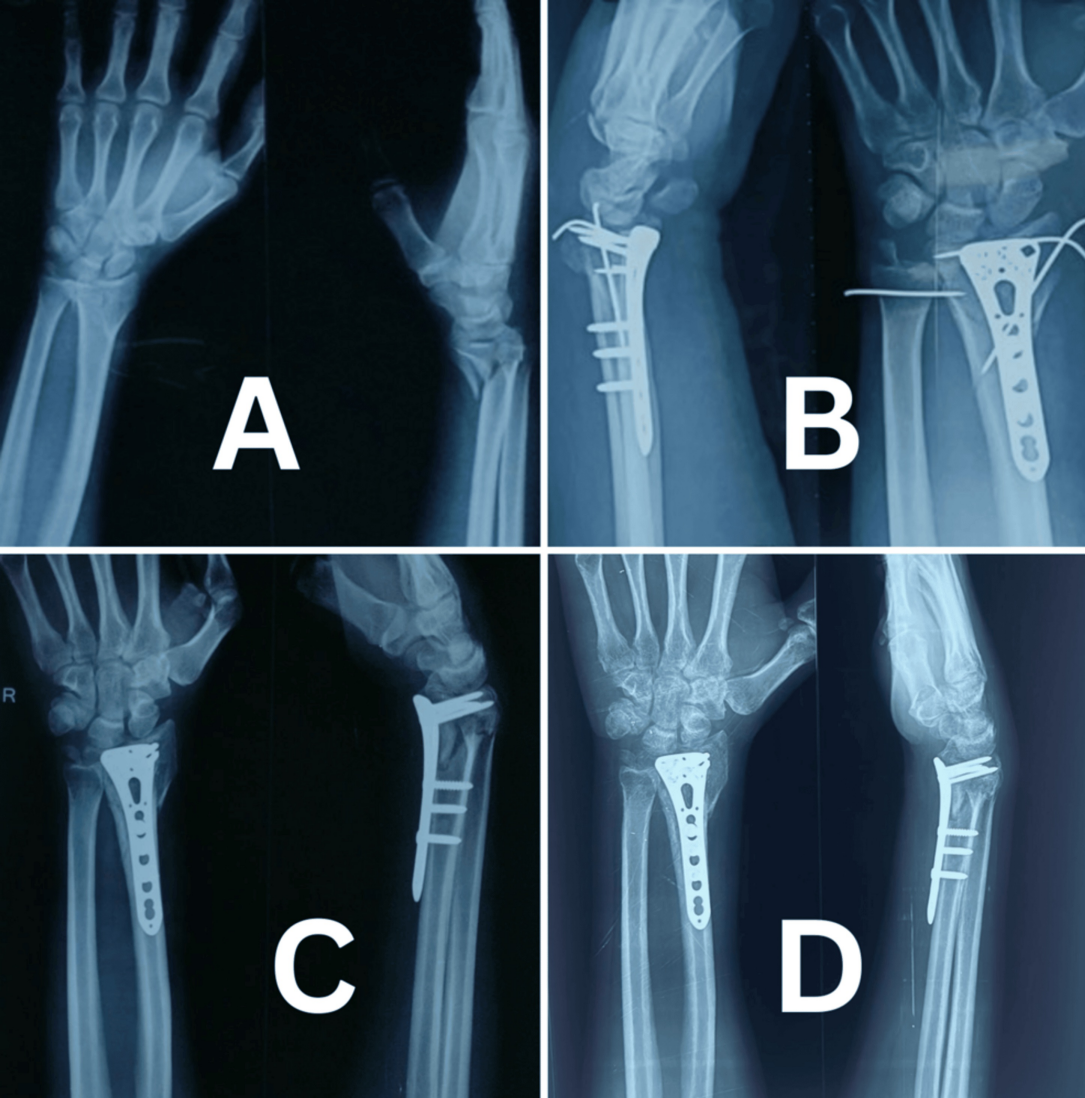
Case 1 wrist X-rays (A) Preoperative anteroposterior and lateral wrist X-rays showing a distal radius fracture. (B) Postoperative anteroposterior and lateral wrist X-rays showing fracture fixation. (C) Three-month follow-up after K-wire removal. (D) Six-month follow-up showing united fracture and congruent joint lines.
